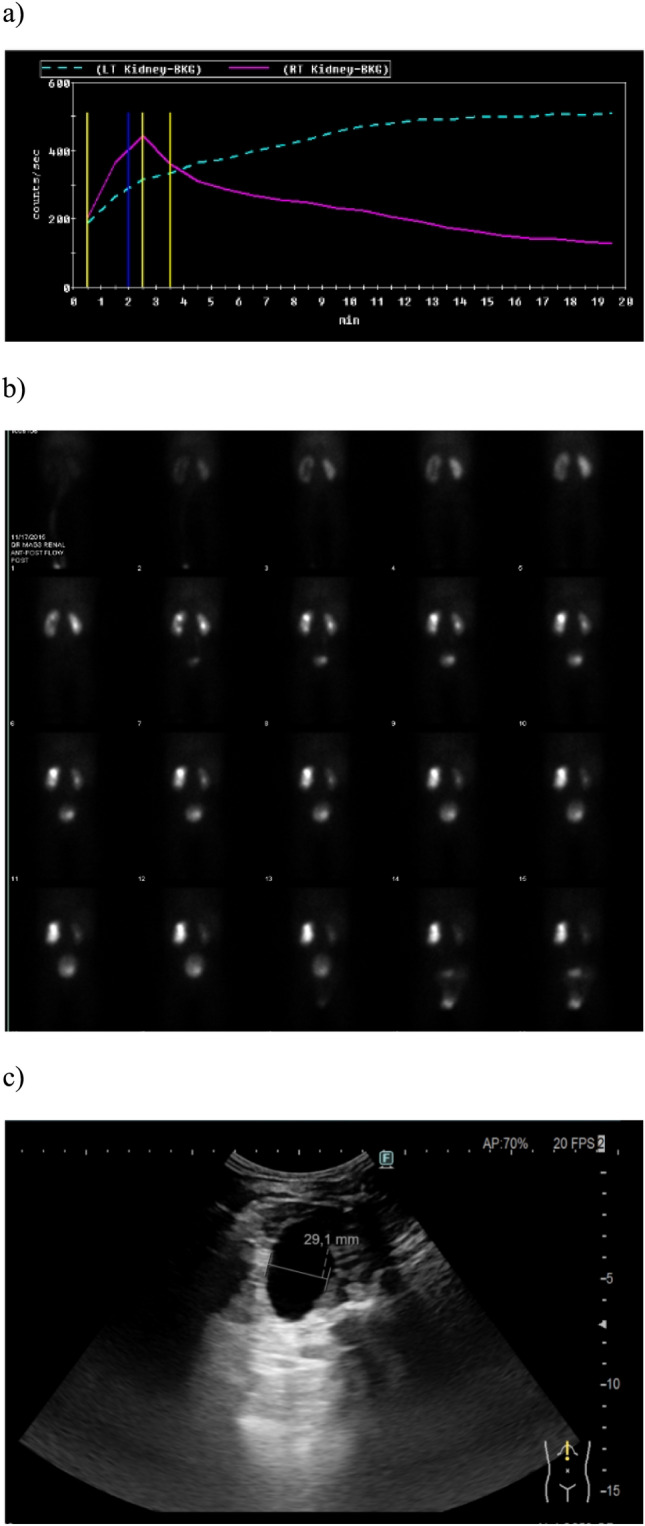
a, b Mercaptoacetyltriglycine-3 (*MAG-3*) scan and c ultrasonographic images of anteroposterior pelvic diameter (*APPD*) measurement on transverse axis in a patient with ureteropelvic junction obstruction (*UPJO*)
